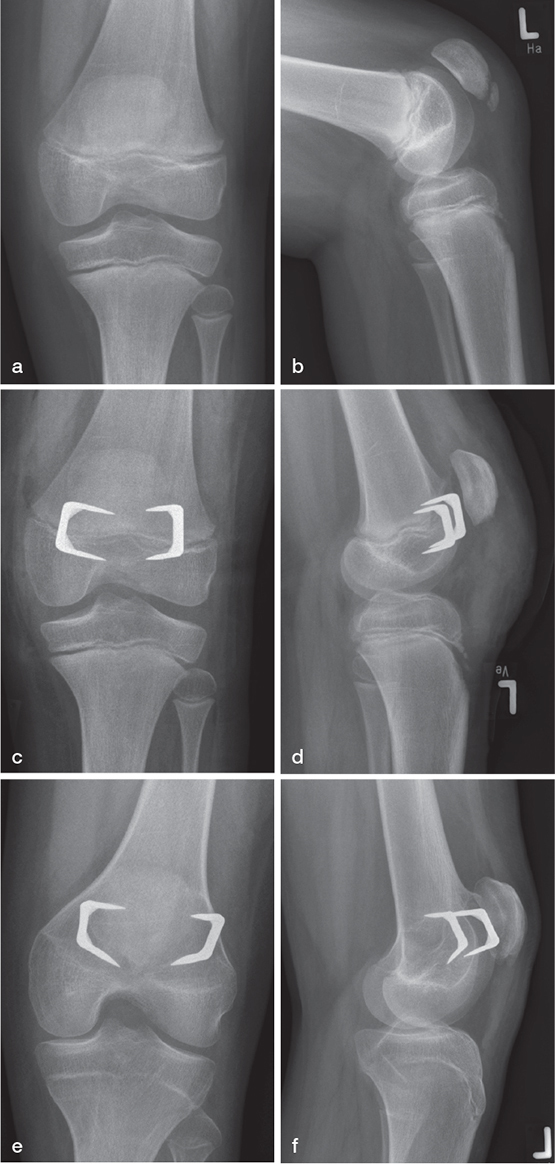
10-year-old boy with myelomeningocele. Before (a, b) and after (c, d) anterior distal femoral hemiepiphysiodesis. Initial radiographs (a, b) show an avulsion fracture of the lower patellar pole in addition. The small patellar fragment was also resected during surgery (c, d). Radiographs taken 3 years after hemiepiphysiodesis and before the implants were removed (e, f). Flexion deformity improved from 10° extension defi ciency to 0°.
